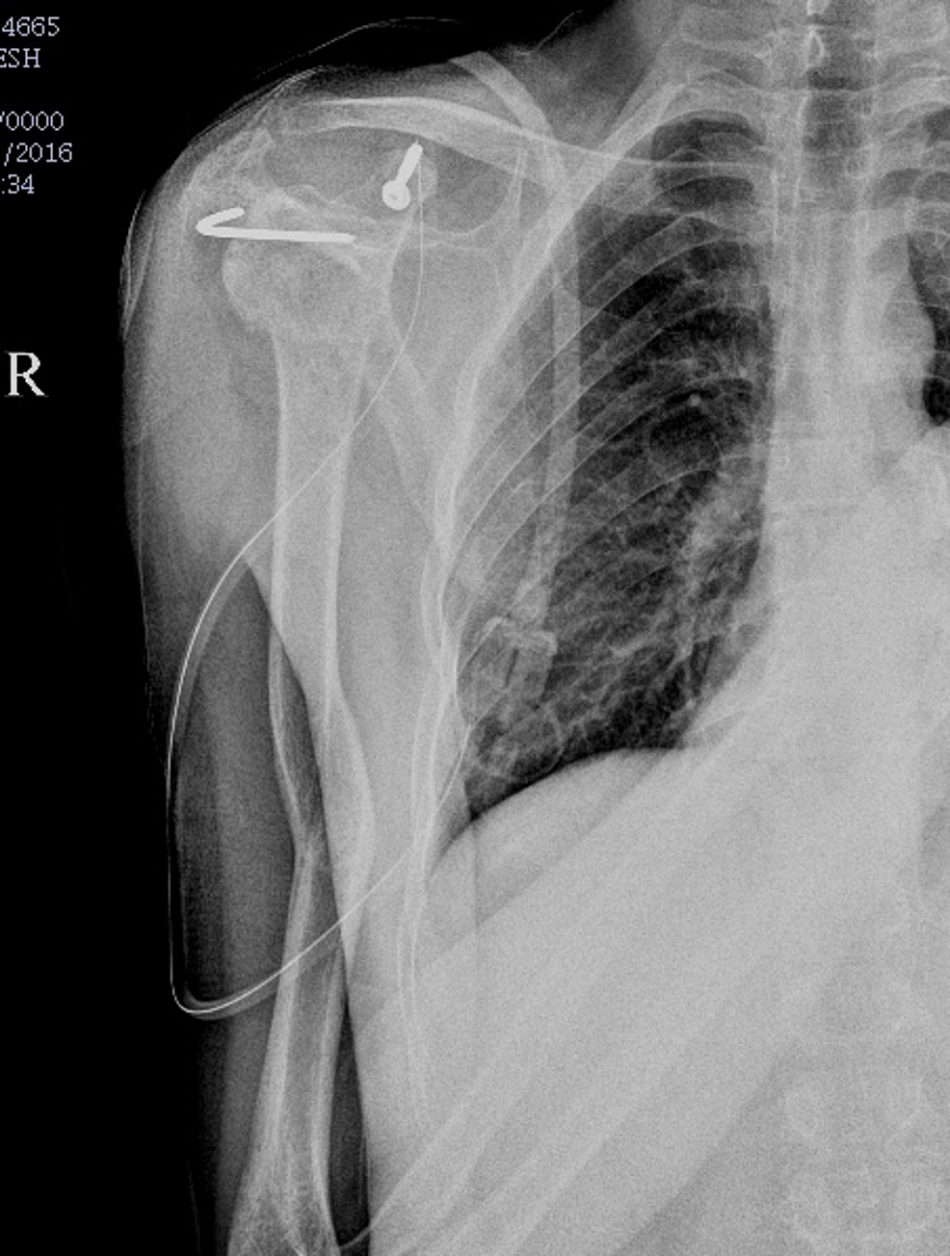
Immediate post-operative X-ray.
